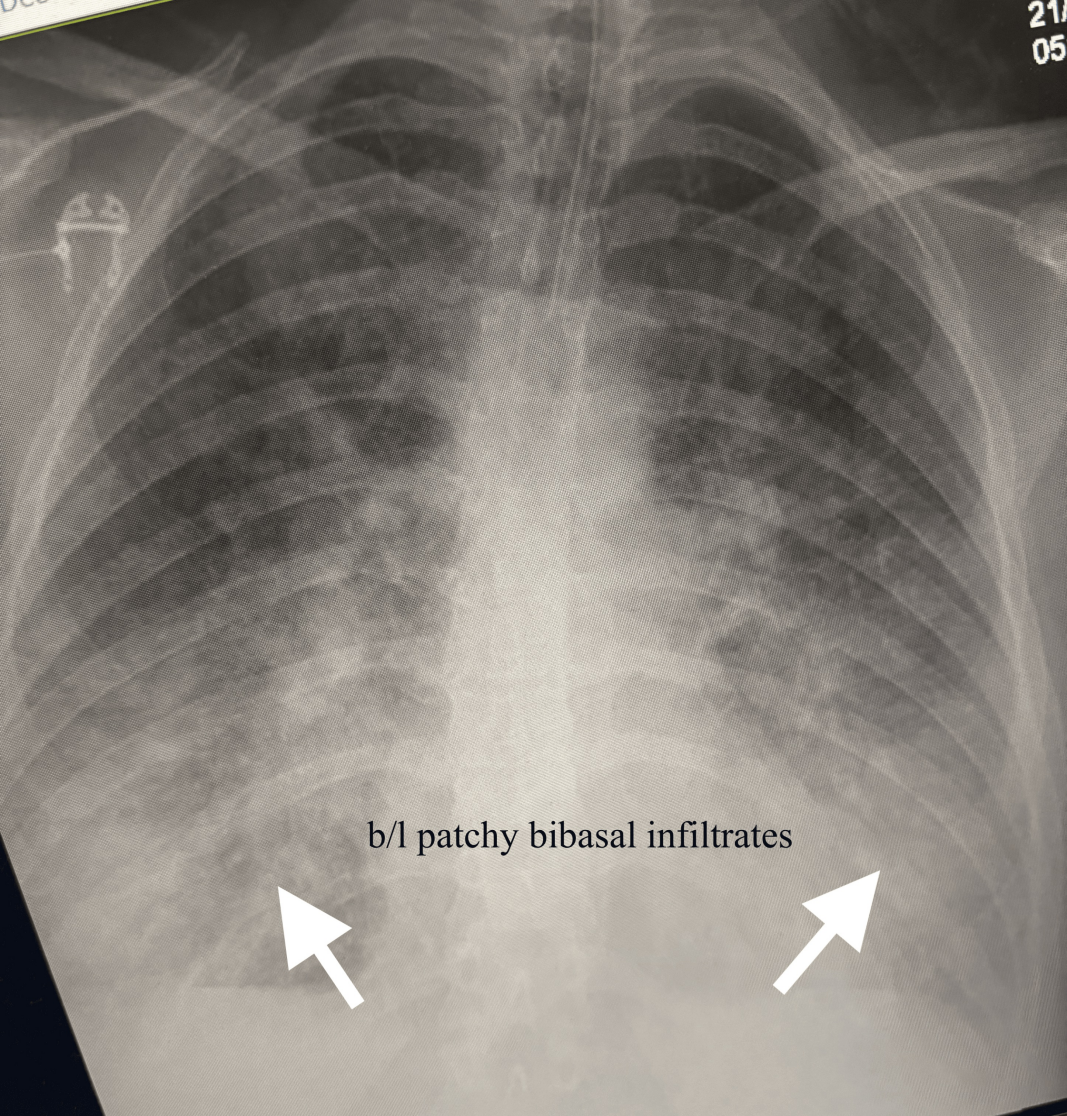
Chest X-ray showing pulmonary oedema
